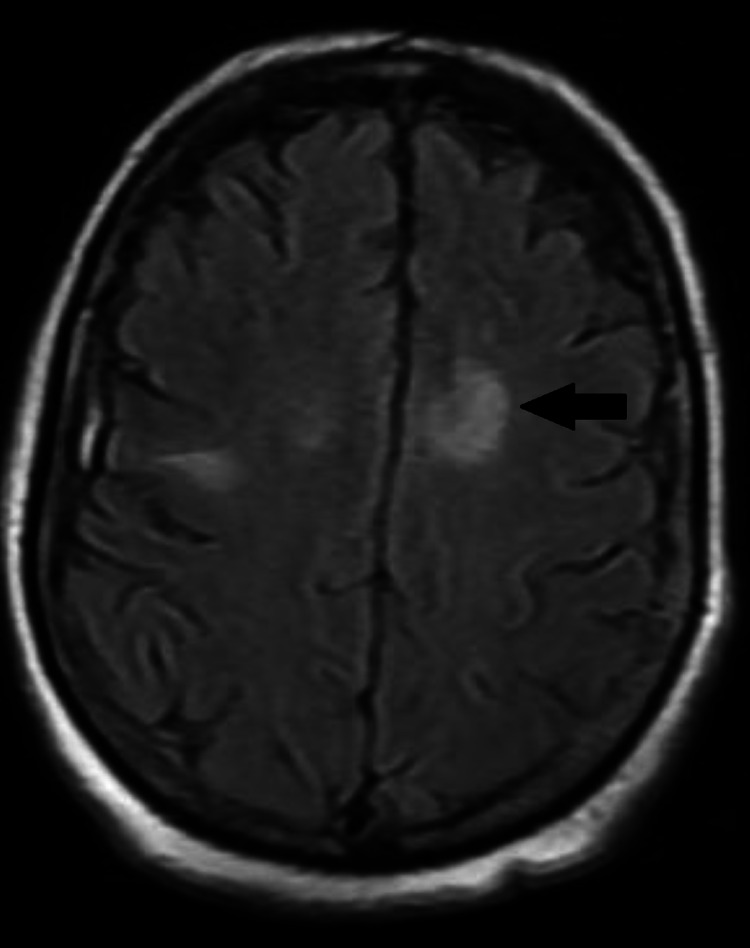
Brain MRI with and without contrast showing confluent T2 hyperintense signals (black arrow) in the bilateral centrum
